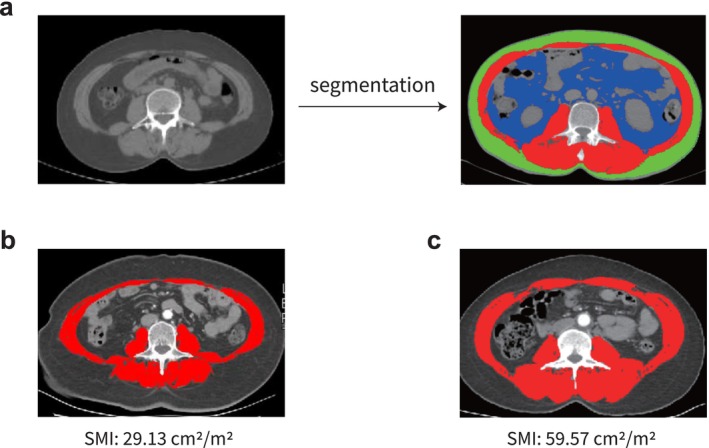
Measurements of body composition parameters. The skeletal muscle volume area (SMA), subcutaneous adipose tissue area (SATA), and visceral subcutaneous adipose tissue area (VATA) were evaluated from a single image at the third lumber vertebra (L3). Analysed CT scans was obtained within 30 days before surgery. The different abdominal tissues were segmented according to their characteristic radiodensity ranges. Skeletal muscle index (SMI), subcutaneous adipose tissue index (SATI), and visceral adipose tissue index (VATI) was calculated by dividing by the square of the patient's height (m^2^). (a) The SMA, SATA and VATA are displayed in red, green and blue, respectively. (b) Example: A 72‐year‐old woman with less skeletal muscle tissue. (c) Example: A 56‐year‐old man with more skeletal muscle tissue.
